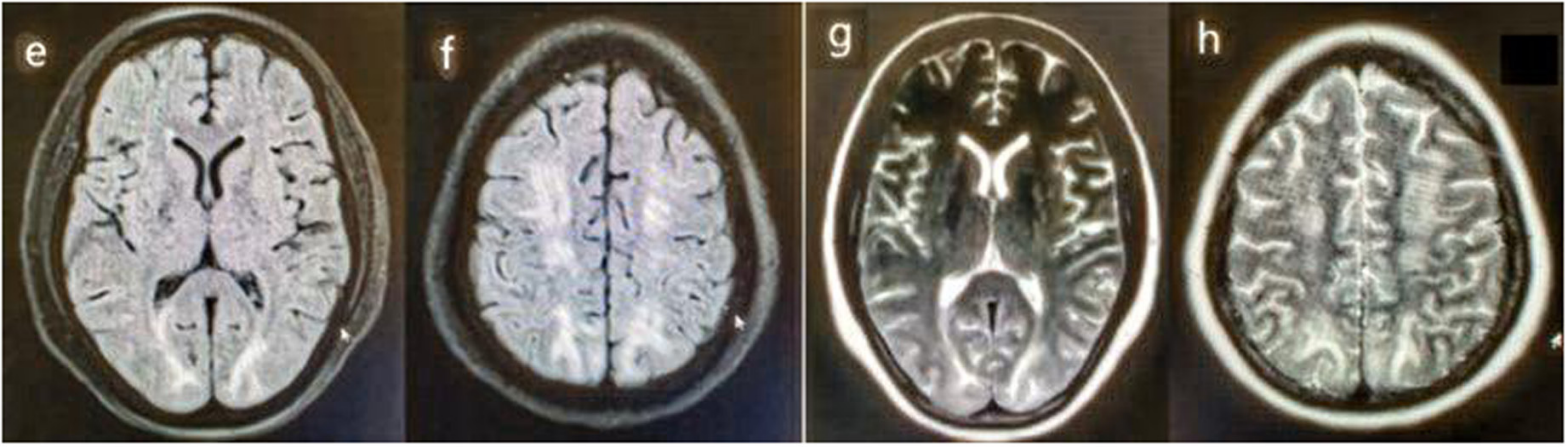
(E, F) Hyperintensities in bilateral occipital in FLAIR, (G, H) Hyperintensities in bilateral occipital and frontal in FLAIR and T2.
